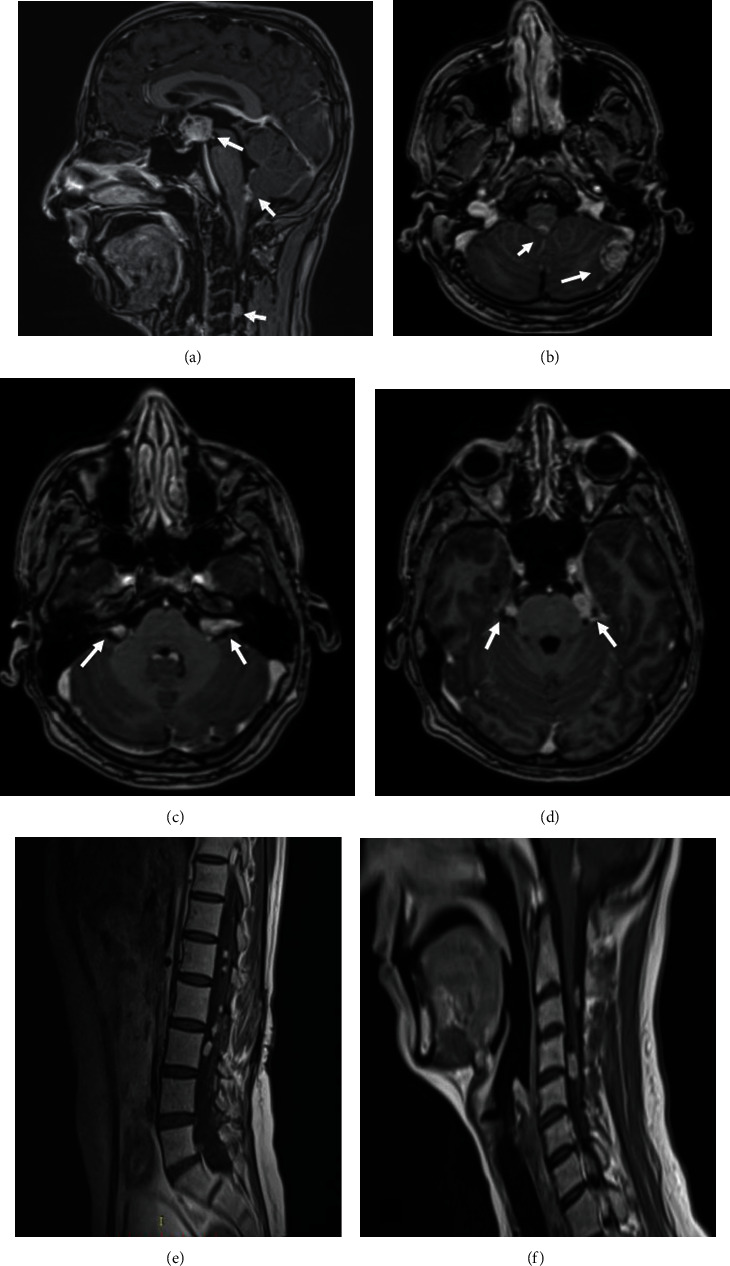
Multisequential multiplanar magnetic resonance images of the brain and the spine with and without contrast. Sagittal (a) and axial (b–d) contrasted T1 brain images and sagittal cervical and lumbar spine (e, f) T1 images reveal an increase in the size of the suprasellar tumor (a) and an increase in the number and size of the meningeal nodular masses in the brain (b–d) and the spine (e, f).
